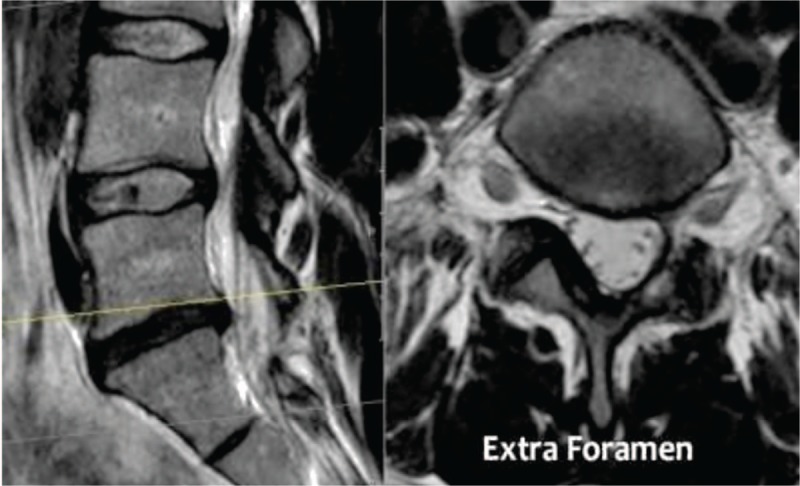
Open lumbar discectomy with pedicle screw fixation. (A) Mid line incision. (B) Image intensifier radiographs of pedicle screw insertion point anterior-posterior view. (C) Intraoperative pedicle screw fixation. (D) Removal of disc materials.
